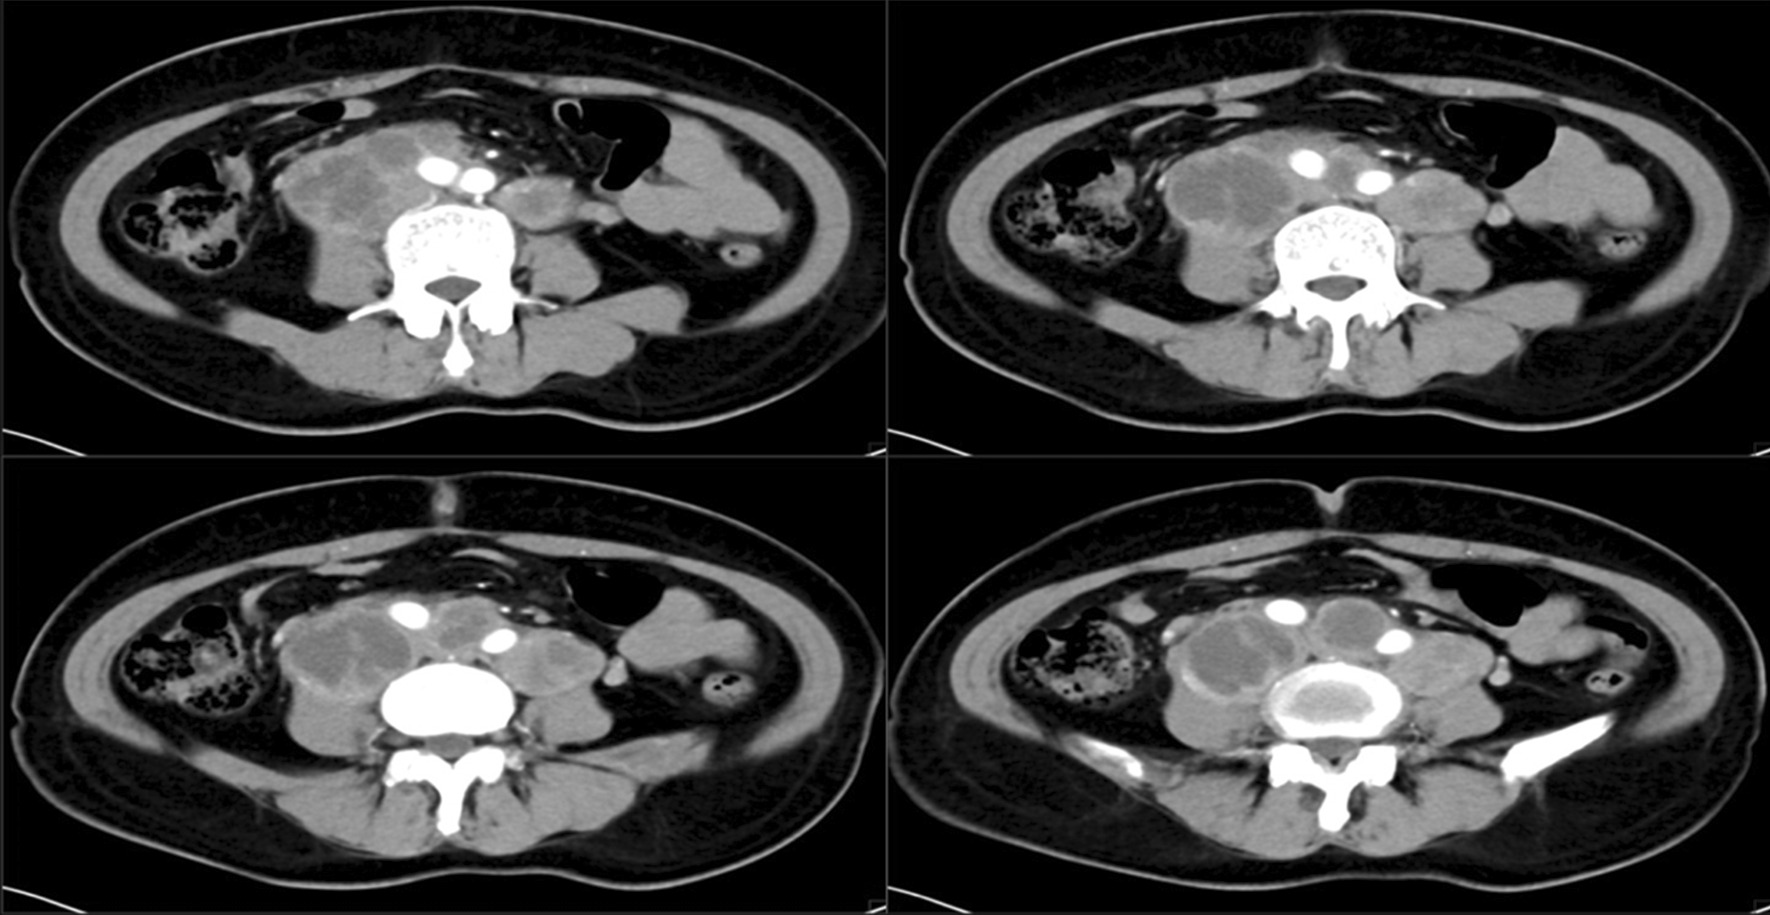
Computed tomography (CT) of the endometrial. Multiple enlarged lymph nodes of varying sizes are seen in the retroperitoneum, adherent to and surrounding the retroperitoneal vessels
